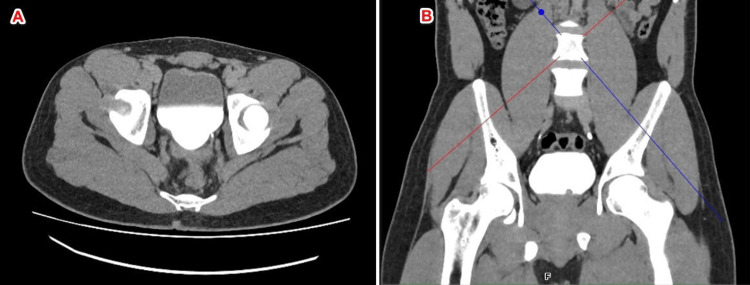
Axial (A) and coronal (B) CT images during the excretory phase (delayed images) showing a sessile filling defect measuring 16 mm in greatest diameter, arising from the left posterolateral bladder wall This lesion, suggestive of a schistosomal pseudotumor, was located on a previously calcified and altered bladder wall.
